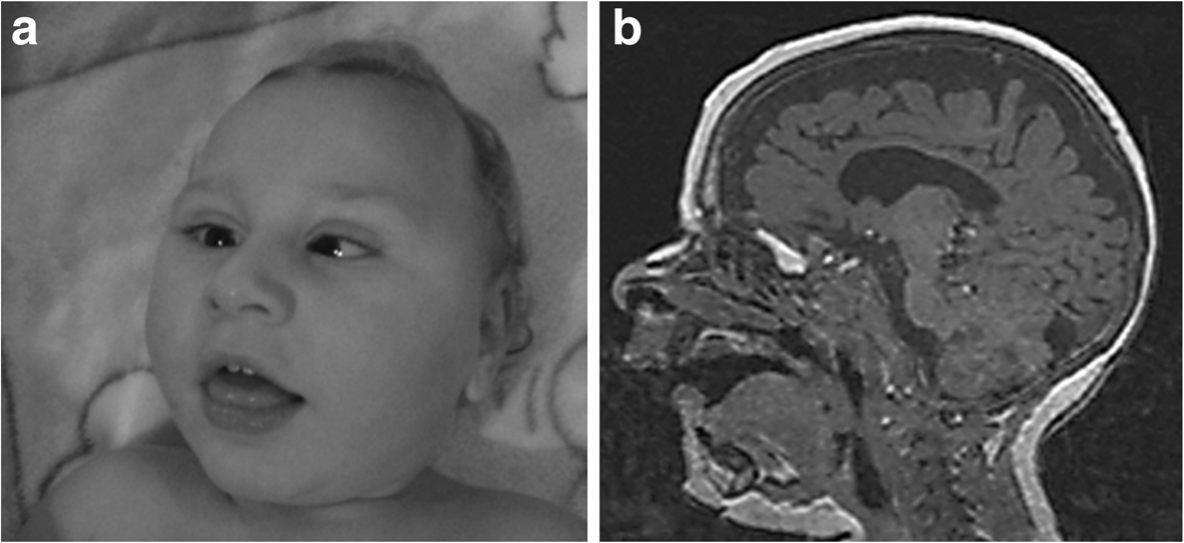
Clinical features and brain MRI of patient 3 at the age of 12 months. Note the important microcephaly, facial hypotonia, retrognathia and strabismus (a). MRI shows global cerebral and cerebellar atrophy (b).
